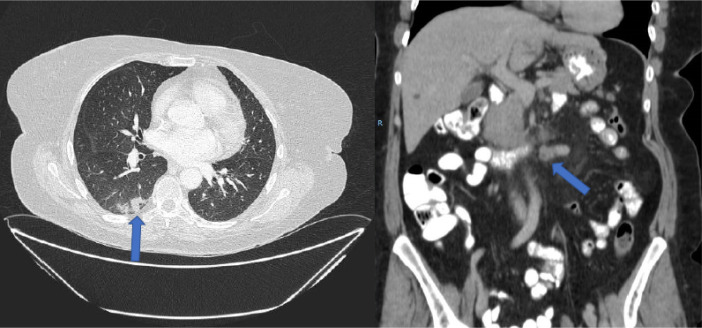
CT prior to the start of treatment with TAS-102. Pulmonary metastasis (blue arrow) and retroperitoneal lymph node involvement are observed. Complete hepatic response is maintained. R: right side of the patient
